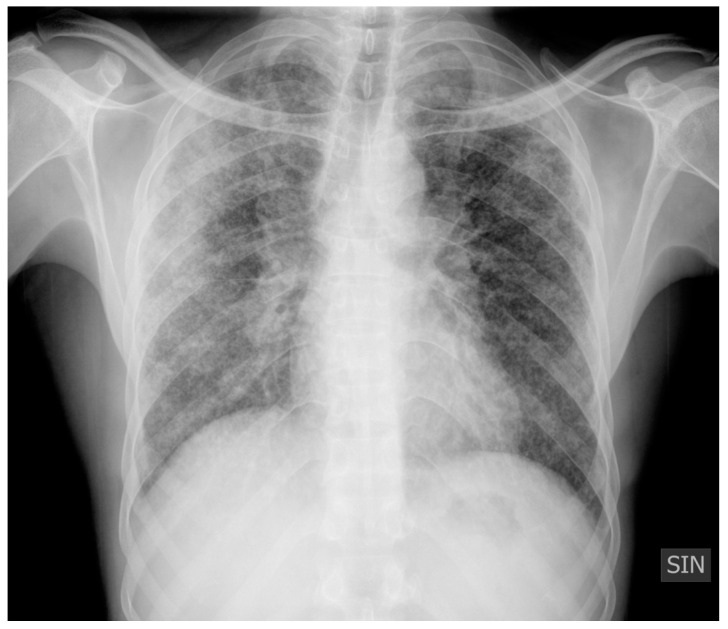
Chest X-ray, AP, showing a range of pulmonary parenchymal abnormalities: multiple micronodules with a peribronchovascular distribution, reticulonodular opacities, right lung consolidation, and symmetric hilar lymphadenopathy. According to Scadding classification, the X-ray in the Figure can be classified as stage II (thoracic adenopathy and pulmonary infiltrates), as in 25–65% of patients.
